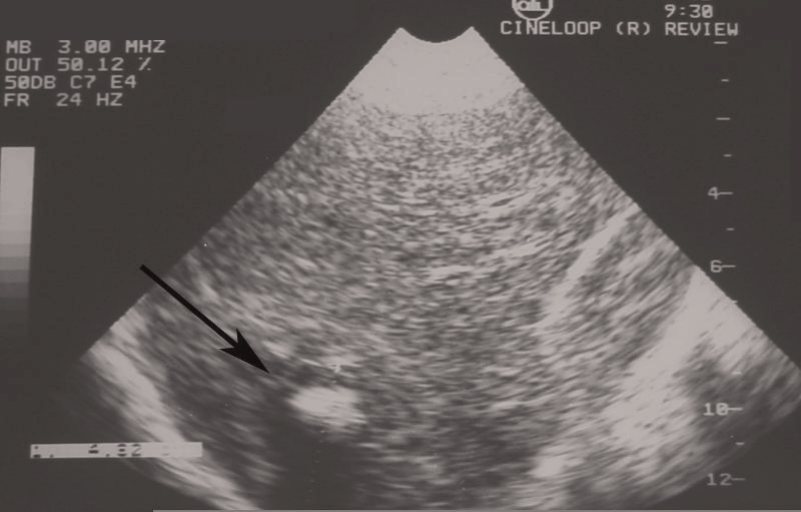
Ultrasound of the liver reveals a central hyperechoic lesion surrounded by a hypoechoic halo with ill-defined borders.
